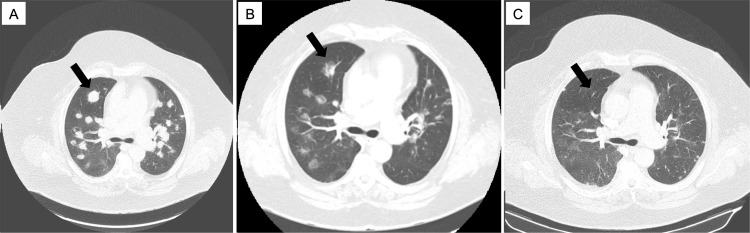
Computed tomography scans showing lung metastatic disease (arrow) at presentation. MEK: mitogen-activated extracellular signal-regulated kinase.
